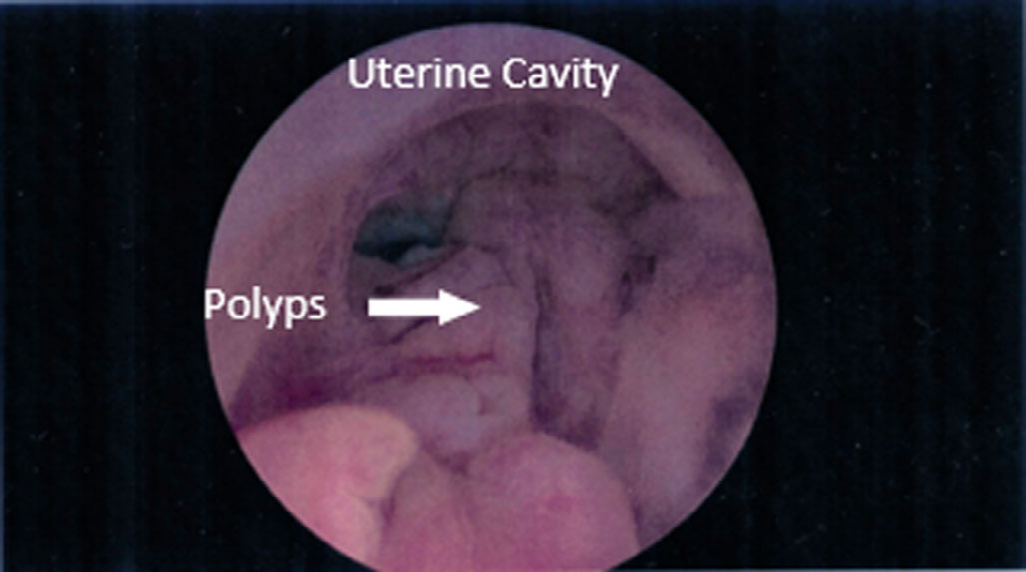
Hysteroscopic image demonstrating multiple benign polyps (arrow) in a female with an endometrial thickening of 7 mm on transvaginal ultrasound. [Colour figure can be viewed at wileyonlinelibrary.com]
